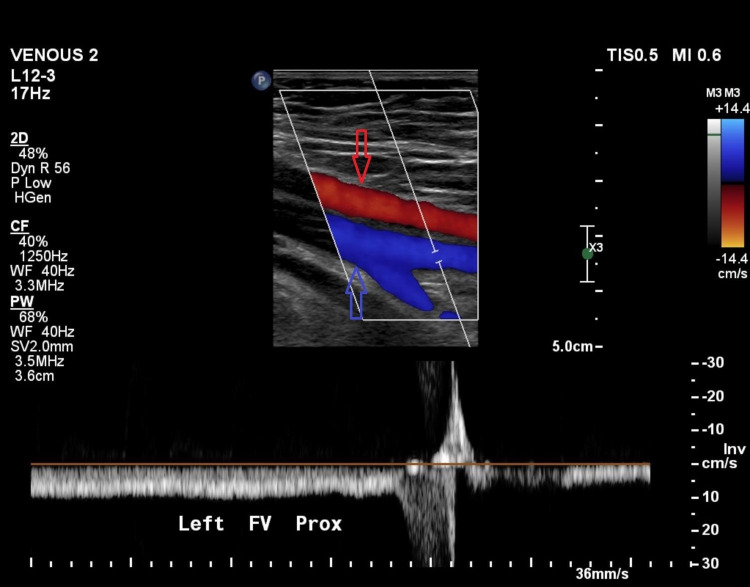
Ultrasound of the pelvis on the six-month follow-up showing patency of the vein (blue arrow) with no residual obstruction, with red arrow showing the overlying artery.
